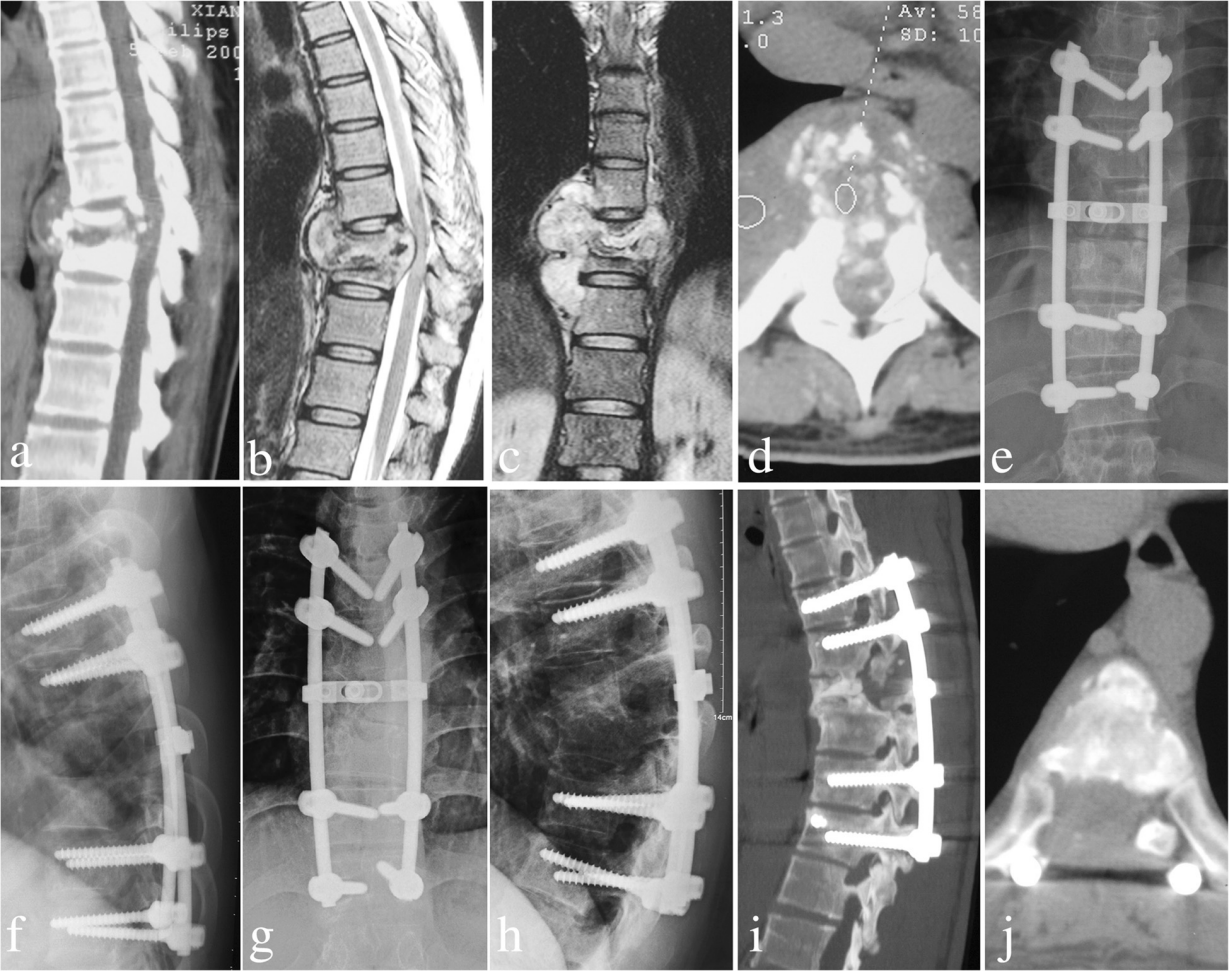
A 27-year-old male with T8/9 lesions was performed by posterior-only approach. a-d The pre-operative imaging data showed T8/9 presented with severe bone destruction, formation of local paraspinal abscess and compression of spinal cord. The postoperative anterior-posterior (e) and lateral X-ray (f) indicated posterior long-segment fixation was in good position. X-ray (g, h) showed good bone fusion and no obvious loss of kyphosis angle in 3 months postoperative. Sagittal and coronal CT-scan (i, j) showed satisfactory bone fusion without presence of complications related to instrumentation and relapse of Pott’s disease at the final follow-up
